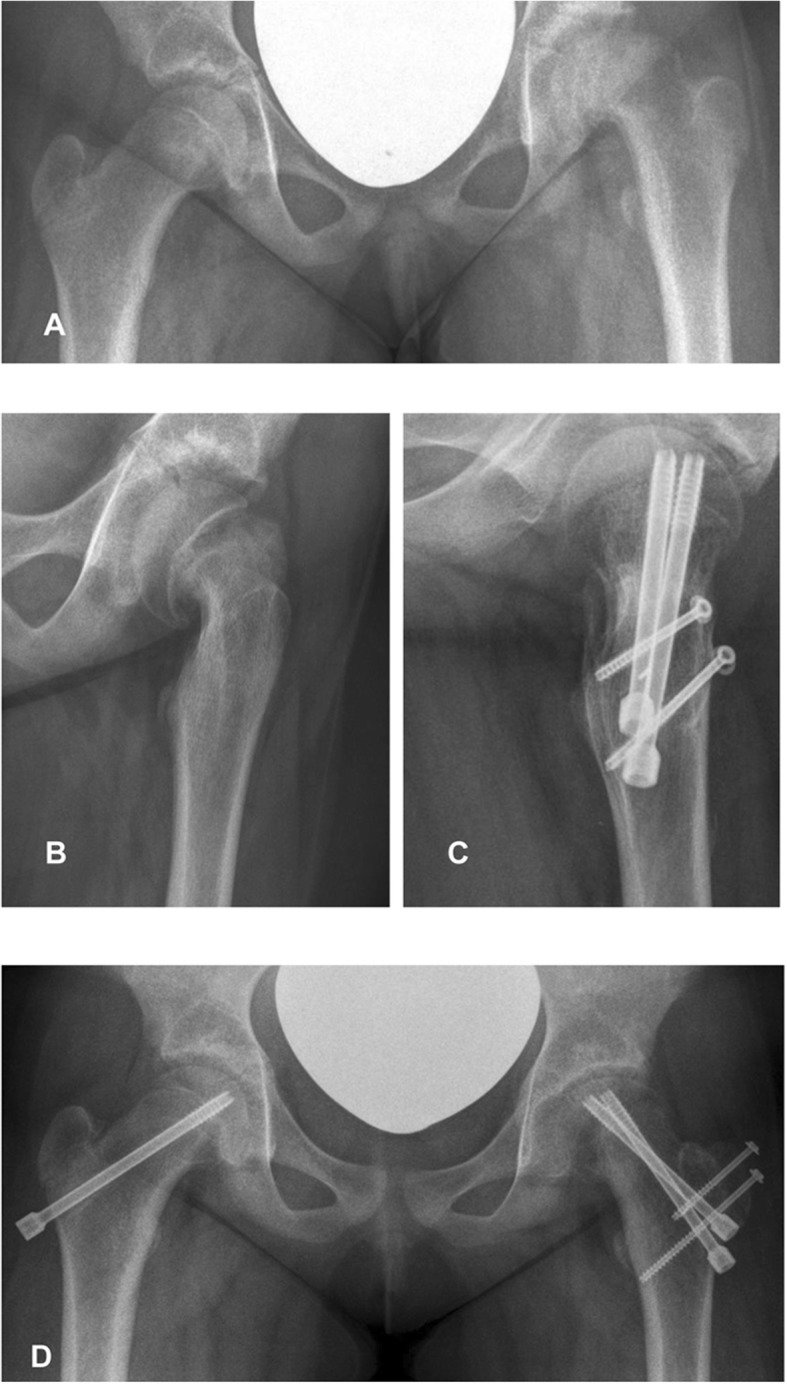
Preoperative (a, b) and 1 year postoperative (c, d) X-rays of a 13-year-old female patient with an acute on chronic SCFE with an SSA of 65°
